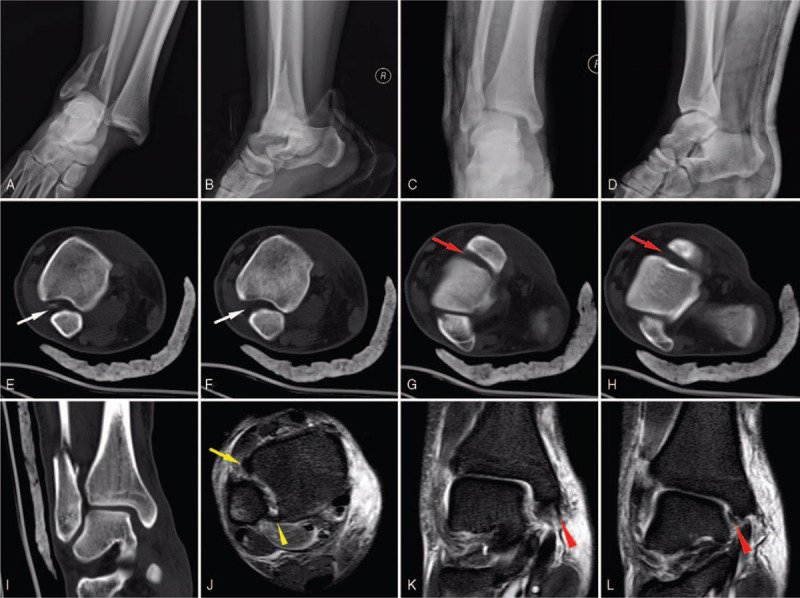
The preoperative imaging materials. (A,B) Anteroposterior and lateral X-ray image of injured ankle immediately. (C,D) Anteroposterior and lateral X-ray image of injured ankle after manual reduction and cast immobilization. (E–H) The different cross sections of CT. The anterior distal tibiofibular syndesmosis (red arrow) and medial ankle mortise (white arrow) were obviously increased. (I) Coronal section of CT shows the asymmetric mortise. (J–L) The MRI views of injured ankle. The anterior-inferior tibiofibular ligament (AITFL) was ruptured (yellow arrow), while the posterior-inferior tibiofibular ligament (PITFL) was intact (yellow triangle arrow). The superior and deep deltoid ligament were also ruptured (red triangle arrow).
